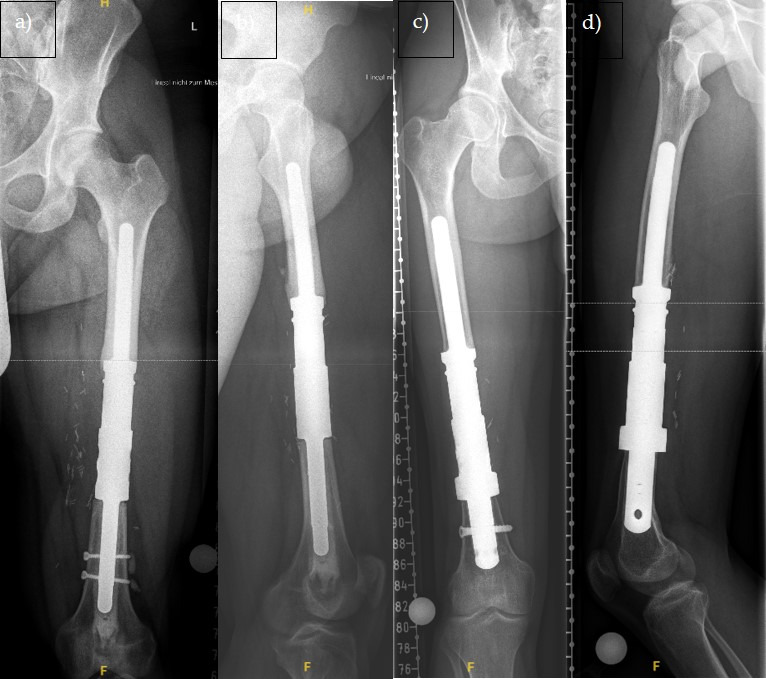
X-ray image in frontal (a) and lateral (b) view of a 34-year-old patient with a left femoral diaphysis implant (standard) and X-ray image in frontal (c) and lateral (d) view of a 26-year-old patient with a left femoral diaphysis implant with ultra short stem (custom made).
